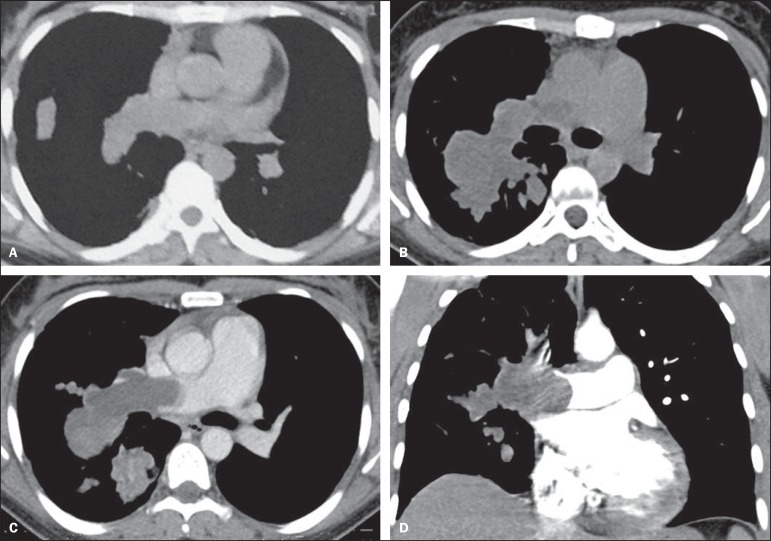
Axial chest computed tomography (A) demonstrating hypodense mass
